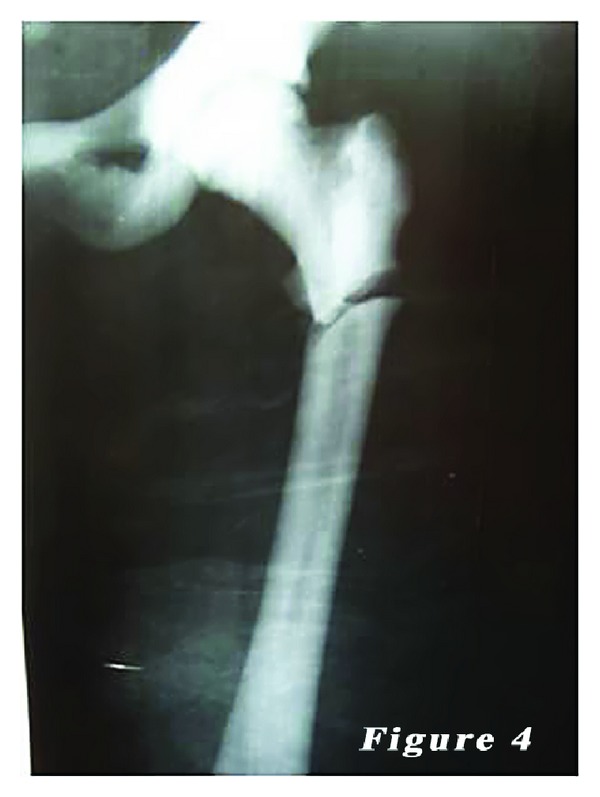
24-year-old female patient (second case) preoperative radiograph of the left femur subtrochanteric fractures.
